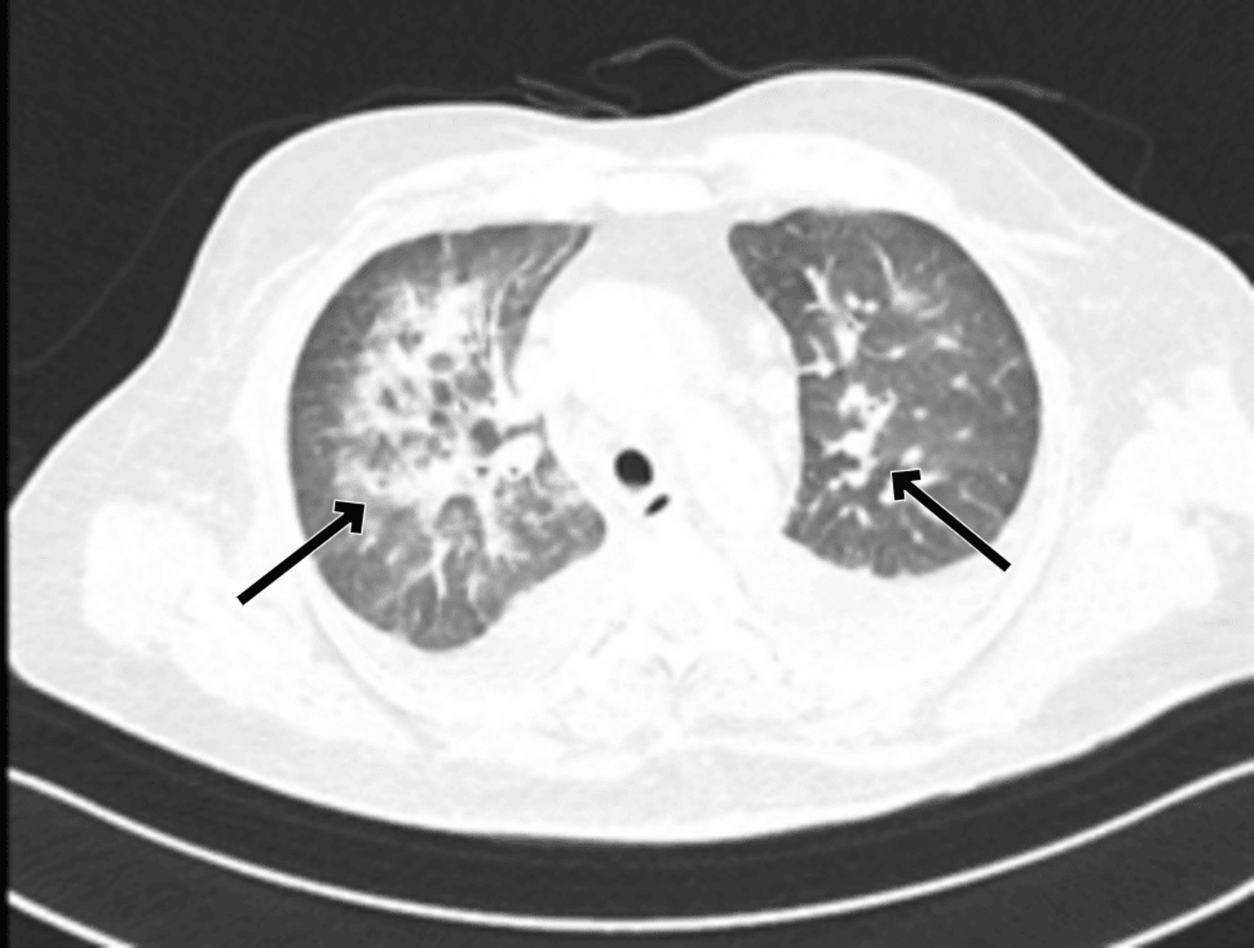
Axial HRCT section of the chest at the level of the bilateral upper lobes, demonstrating confluent ground-glass opacities and consolidation in perihilar and central locations with relative sparing of the lung periphery (arrows). HRCT: high-resolution computed tomography
